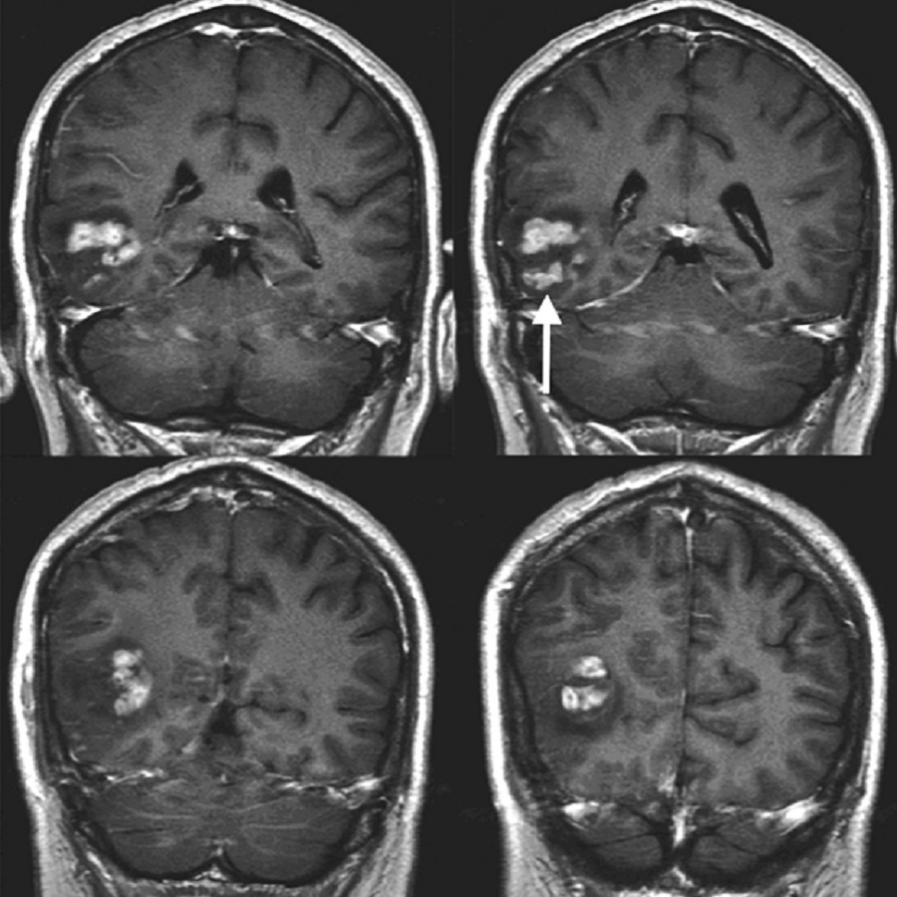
Coronal T1 post-contrast MRI Heterogeneously enhancing mass centered in the right posterior temporo-occipital region involving both the cortex and subcortical white matter with surrounding vasogenic edema (arrow)
